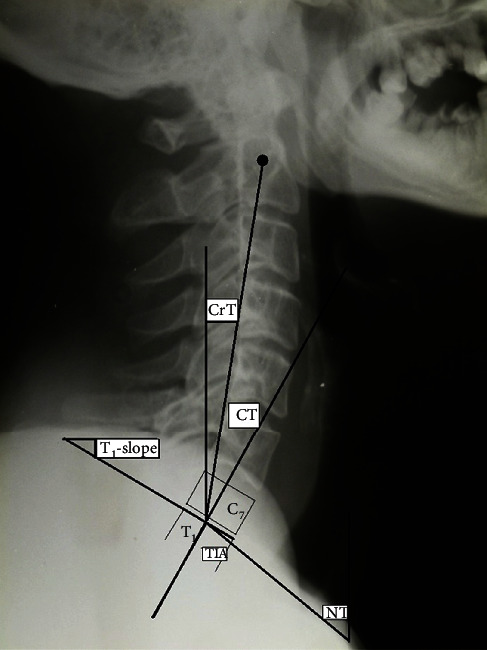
Sagittal roentgenographic parameters on standing lateral skull and cervical roentgenogram. CrT = cranial tilt; CT = cervical tilt; NT = neck tilt; TIA = thorax inlet angle.
